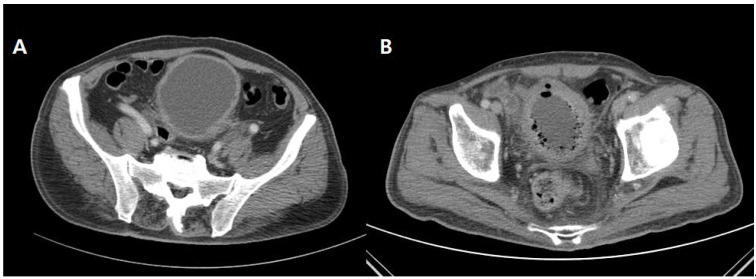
Axial computed tomography (CT) image through the lower pelvis of patients with acute cystitis (A) and emphysematous cystitis (B), with diffuse bladder wall thickening shown in the bladder of the patient with acute cystitis and gas collection within the bladder wall and lumen in the patient with emphysematous cystitis.
